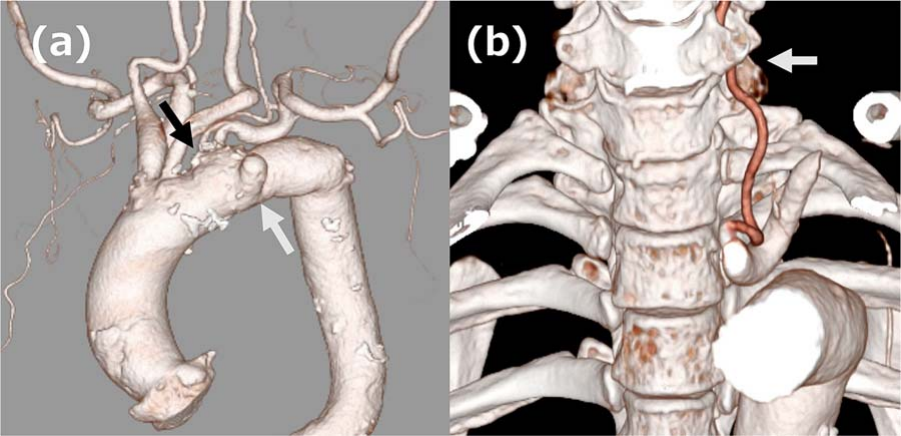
(a) Three-dimensional computed tomography (3DCT) image. The white arrow indicates the aortic aneurysm, and the black arrow indicates ILVA. (b) 3DCT image. The white arrow indicates the entry of ILVA under the transverse process of the vertebra at the sixth cervical vertebra.
